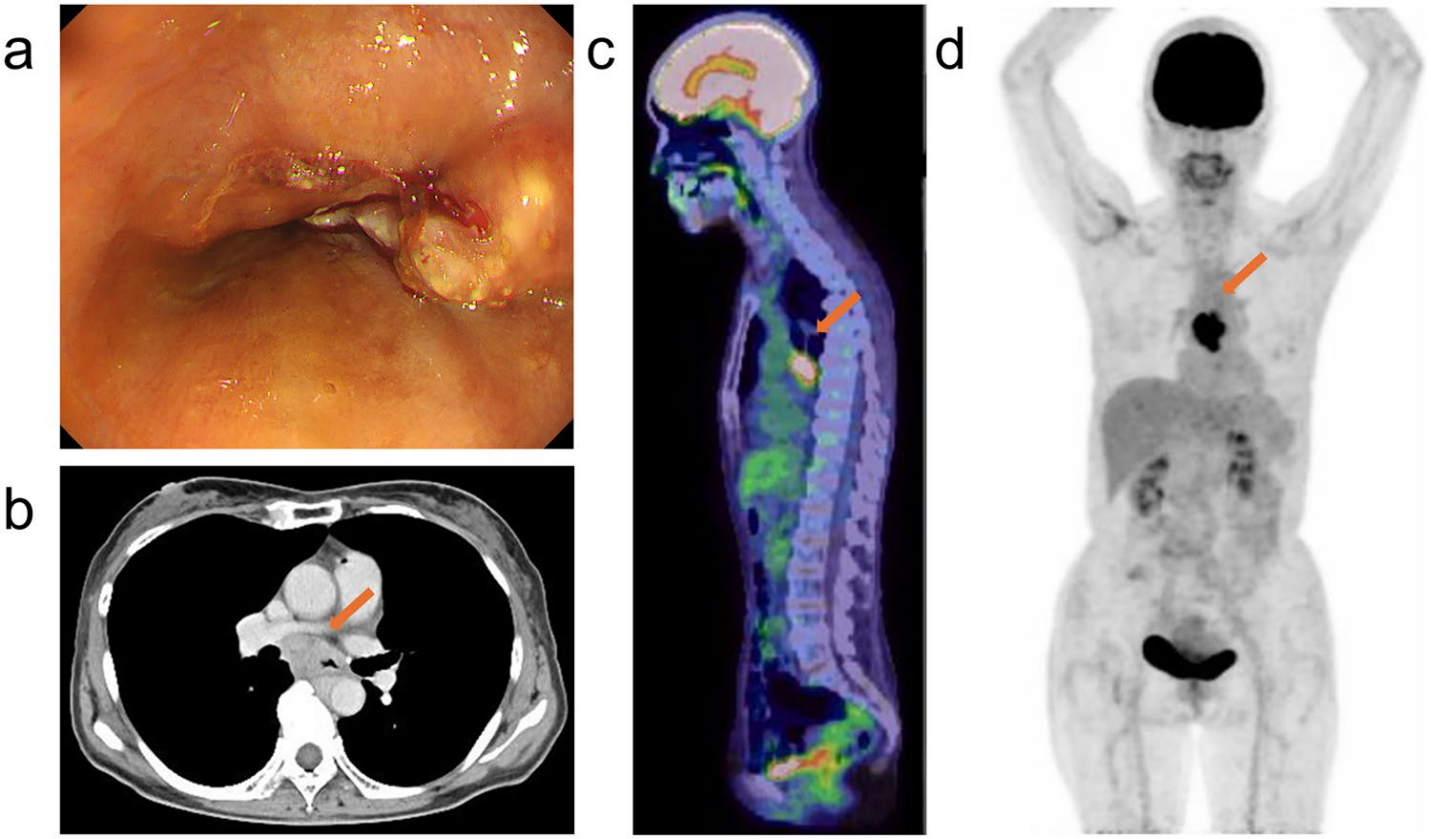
a Upper gastrointestinal endoscopy showed type 1 esophageal cancer in the middle thoracic esophagus. b Iodine contrast CT showed esophageal cancer invading the aorta (indicated by arrow in b; axial CT image). c and d FDG-PET/CT showed accumulation in the primary lesion with mediastinal lymphadenopathies (indicated by arrows in c; sagittal fused PET-CT scan and d; MIP image from PET scan) and no distant metastases. *CT* computed tomography, *FDG-PET* 18F-fluorodeoxyglucose positron emission tomography, *MIP* maximum intensity projection
